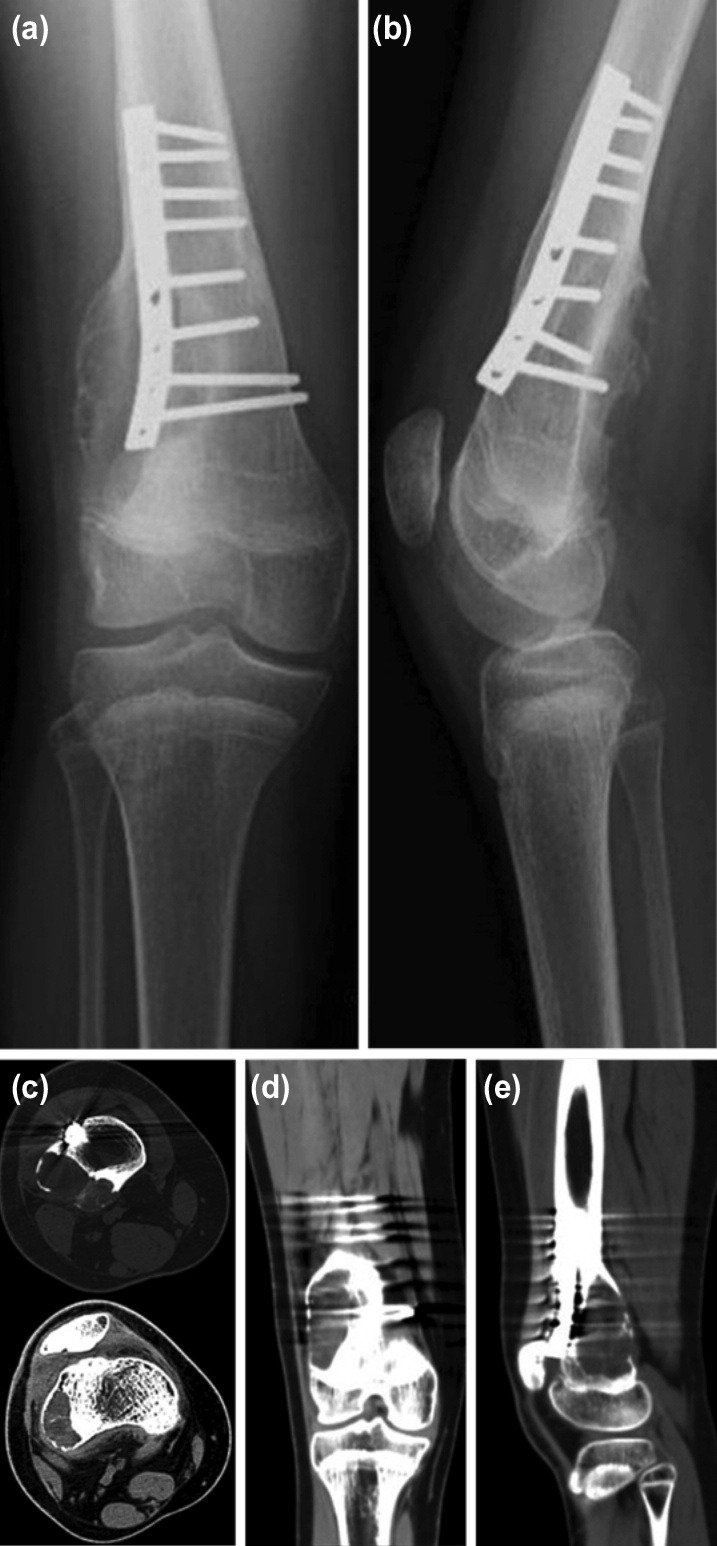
(a) Anteroposterior and (b) lateral radiographs of the right knee at the 2nd year follow-up after femoral derotational osteotomy. Radiographs show a large bone cyst on the distal lateral portion of the right femur. (c) Axial, (d) coronal and (e) sagittal computed tomography scan images of the right knee showing location and expansion of the cyst.
